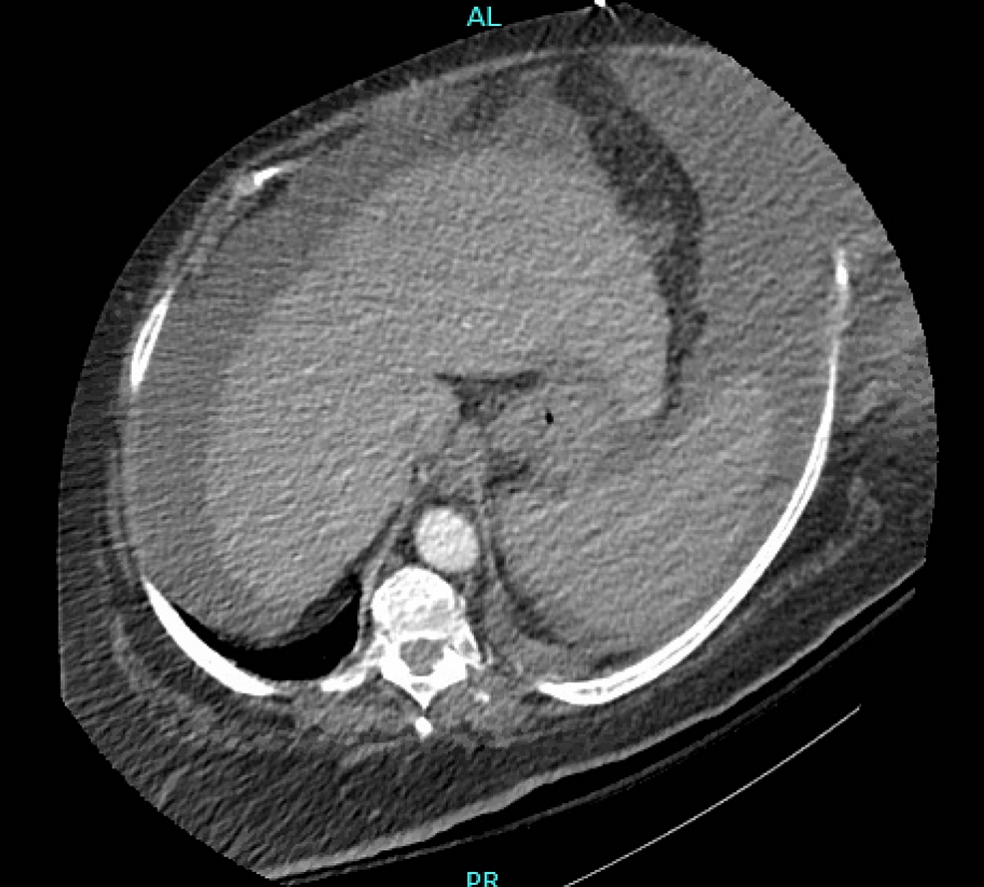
The patient's CT scan of the abdomen demonstrates cirrhotic liver
